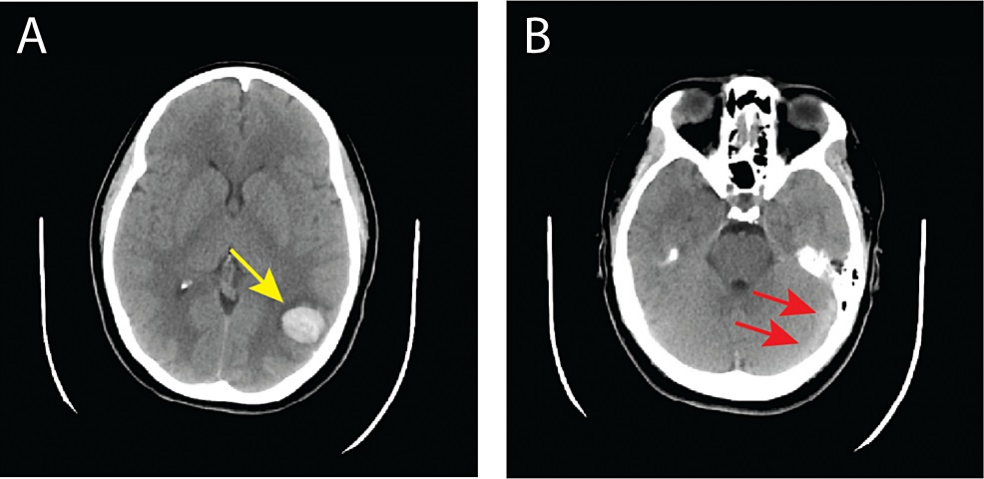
Initial non-contrast CT scan of the head demonstrating an intraparenchymal hemorrhage (A; yellow arrow) due to cerebral venous sinus thrombosis evidenced by the hyperdense left transverse sinus (B; red arrows).
